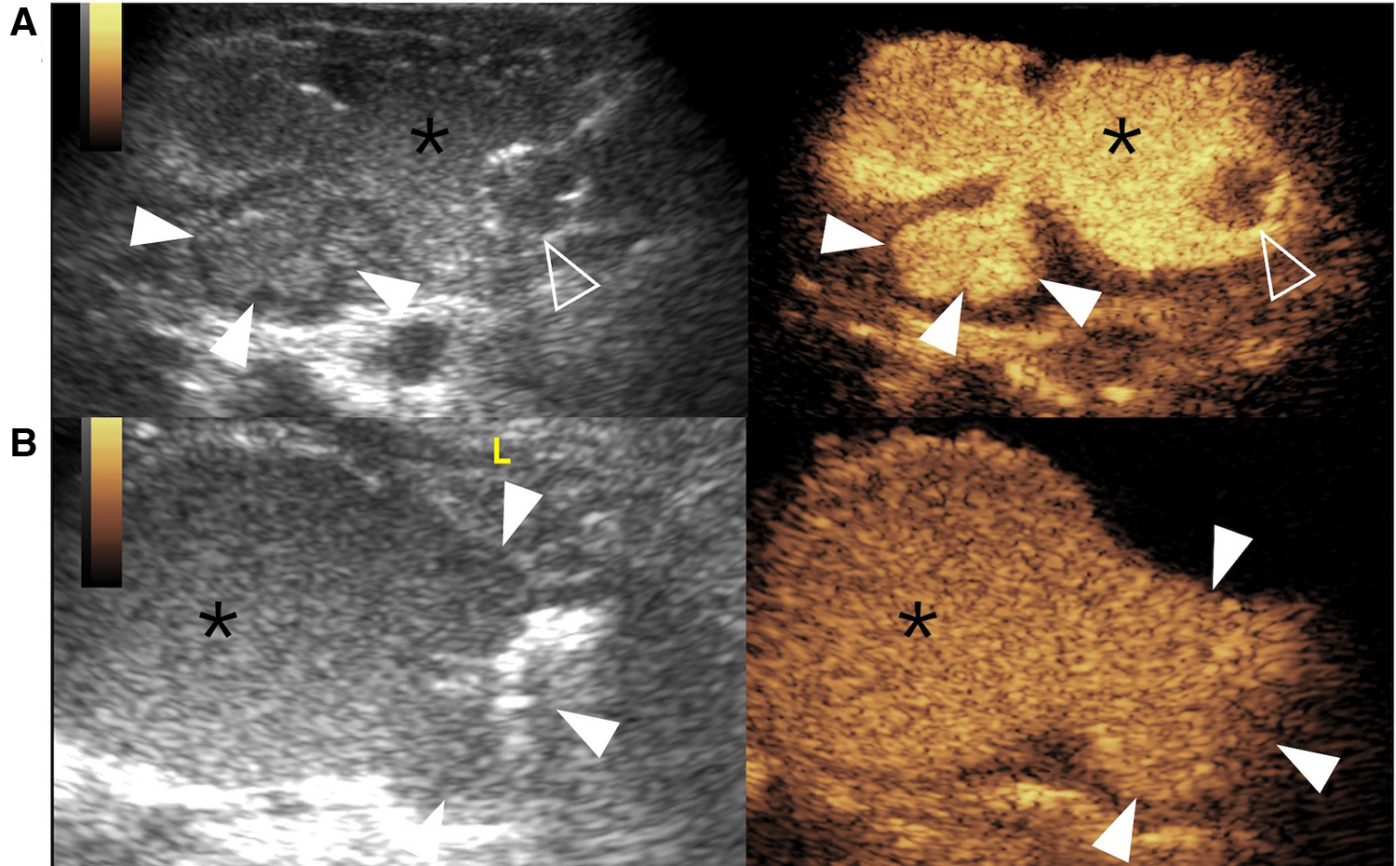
Bladder diverticulum on contrast-enhanced voiding urosonography of the dual-screen mode in a 3-year-old boy (A) and a 1-year-old boy (B). (A) The sagittal plane of the bladder (asterisks) shows a contrast-filled saccular-like diverticulum (solid arrowheads) behind the bladder. The open arrowhead indicates the echogenic balloon. (B) The transverse plane of the bladder (asterisks) shows a large contrast-filled diverticulum (solid arrowheads) on the left of the bladder.
